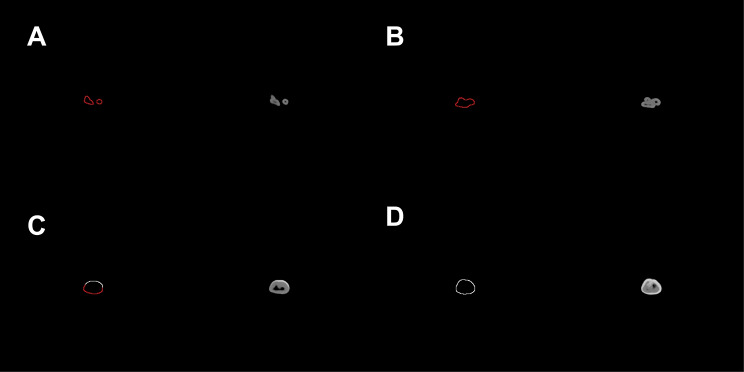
CBCT axial visualization images of isolated tooth roots segmented based on the dynamic threshold method. Images of four axial levels (A-apical 1/3, B-middle 1/3 of the root, C-root-crown junction, and D-crown level) have been selected as representative images. The red color indicates the root, and the white color indicates the crown
